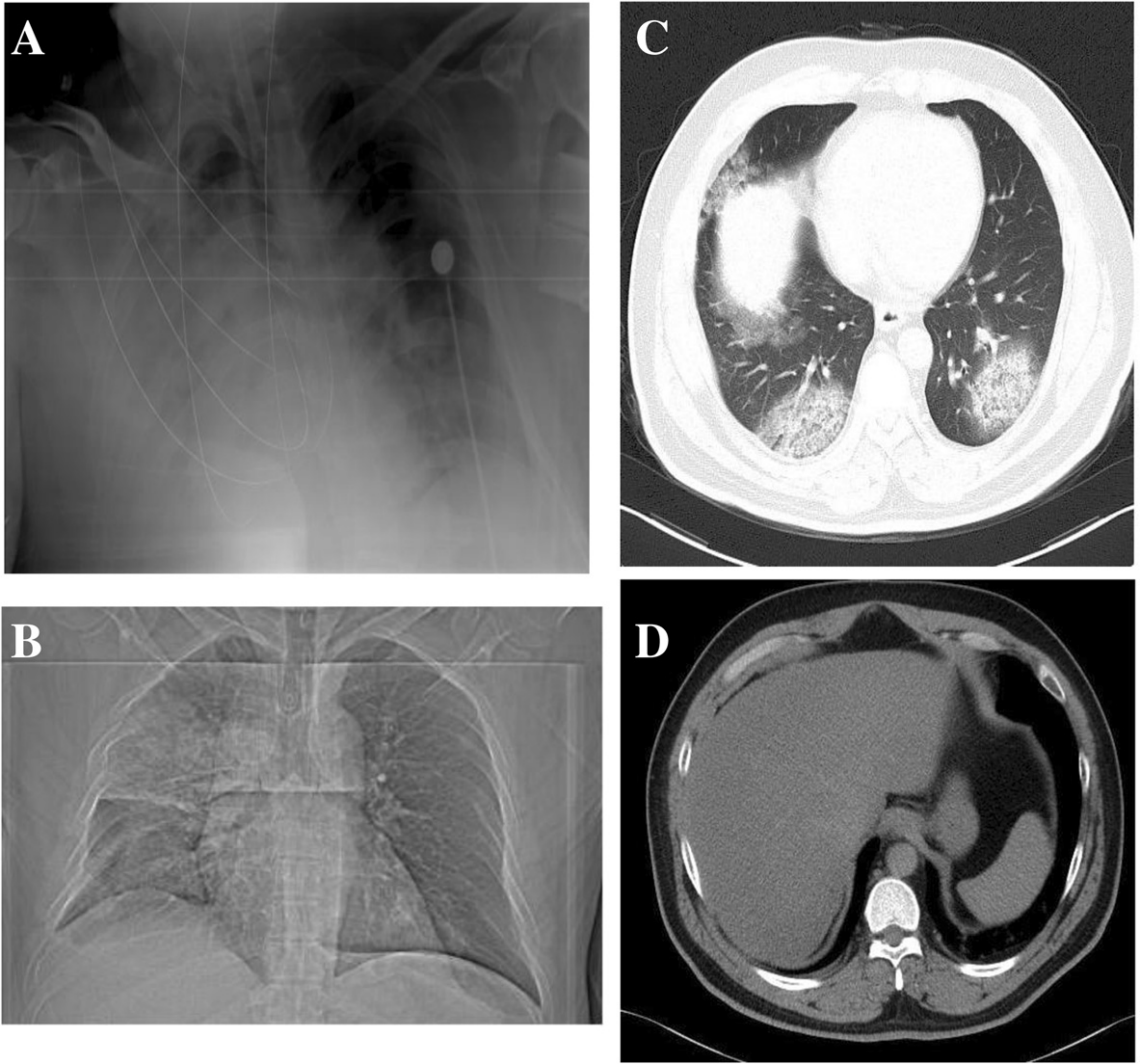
A chest radiograph of the index case is shown in Panel A and B on day 6 after the onset of the illness. A computed tomography scan of the chest of the index case is shown in Panel C and D after the onset of the illness.
